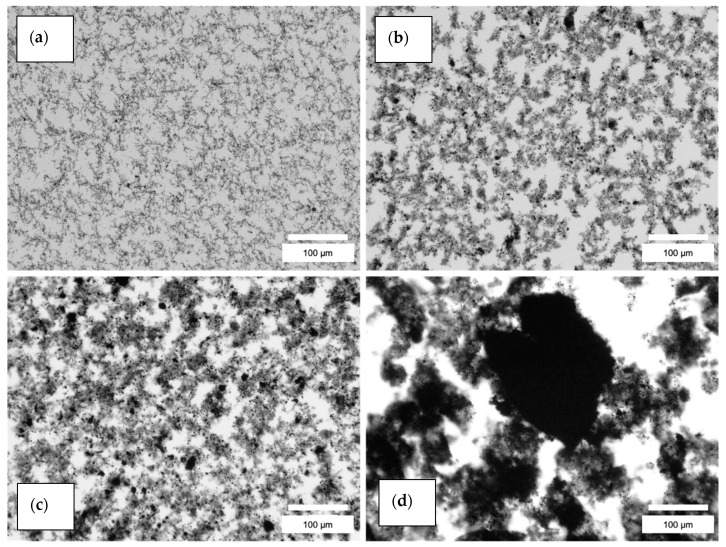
Comparison of different nanofillers dispersed in hardener (ultrasonication—US): (a) 0.5 wt% carbon nanofiber (CNF); (b) 0.5 wt% CNToxi; (c) 0.5 wt% carbon nanotube (CNT); (d) 0.5 wt% CNT semi-dispersed.
